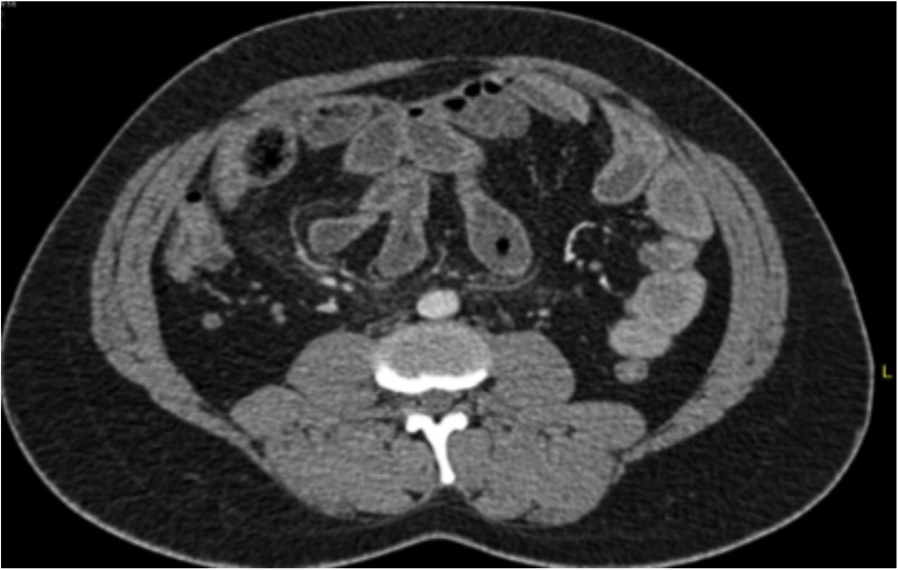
Contrast-enhanced CT scan of the abdomen showing a conglomerate of multiple small-bowel loops seen in the center of the abdomen, surrounded by a thick enhanced saclike structure
